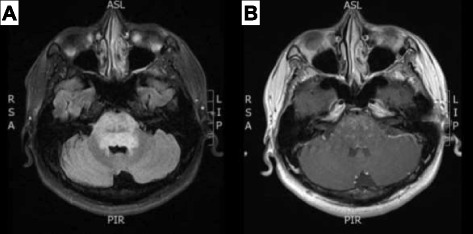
a Fluid-attenuated inversion recovery image shows ill-defined hyperintensity involving the whole pons, which was associated with swelling of the pons. b Postcontrast T1-weighted image shows multiple punctate and curvilinear enhancing lesions in the pons bilaterally
